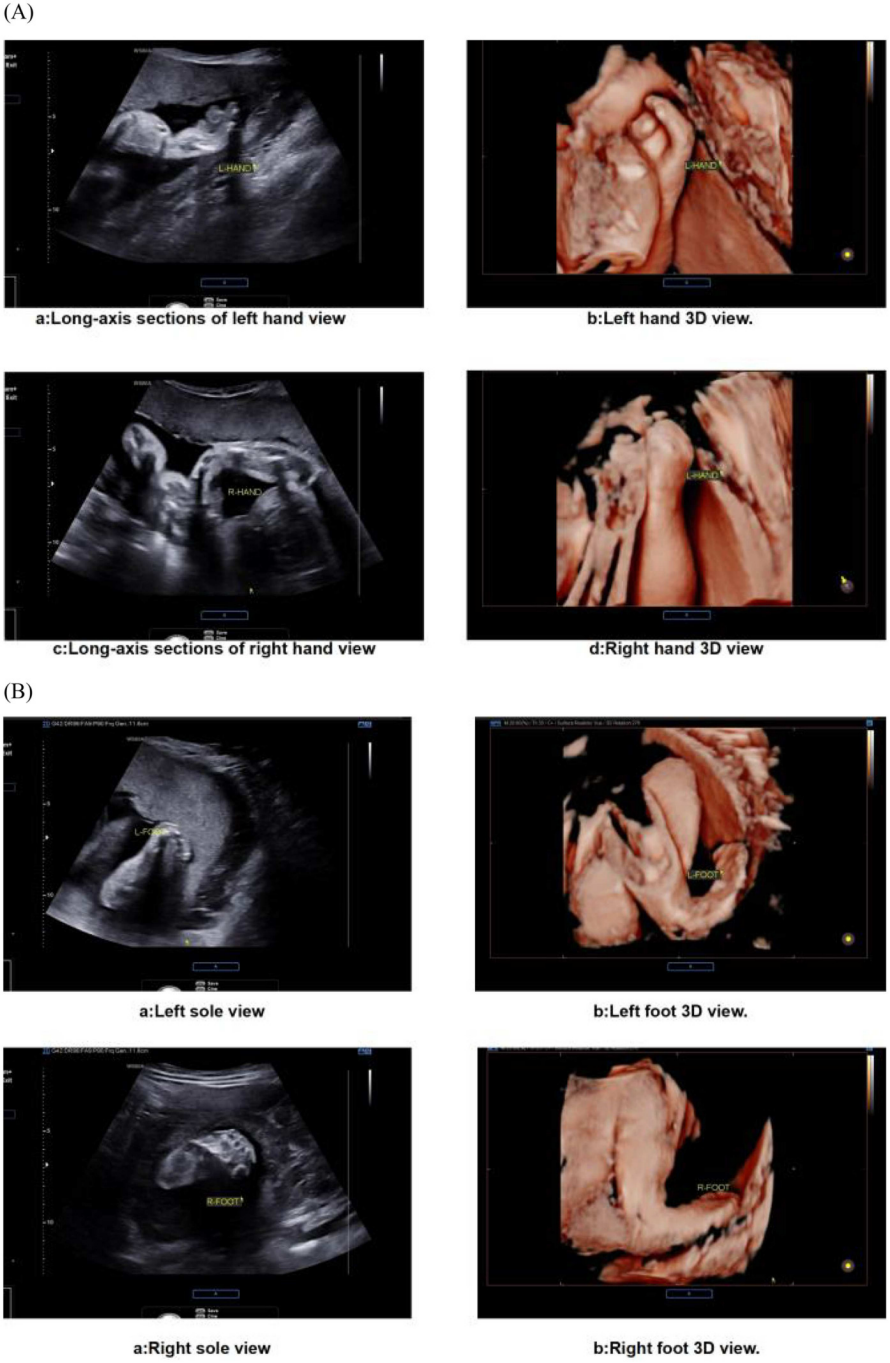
Ultrasound images of fetal extremities in apert syndrome. (A) The presence of an abnormal hand shape, a fixed posture, and an atypical arrangement of the metacarpal bones and phalanges is indicative of the “glove sign”. (B) The feet display a “glove sign”.
